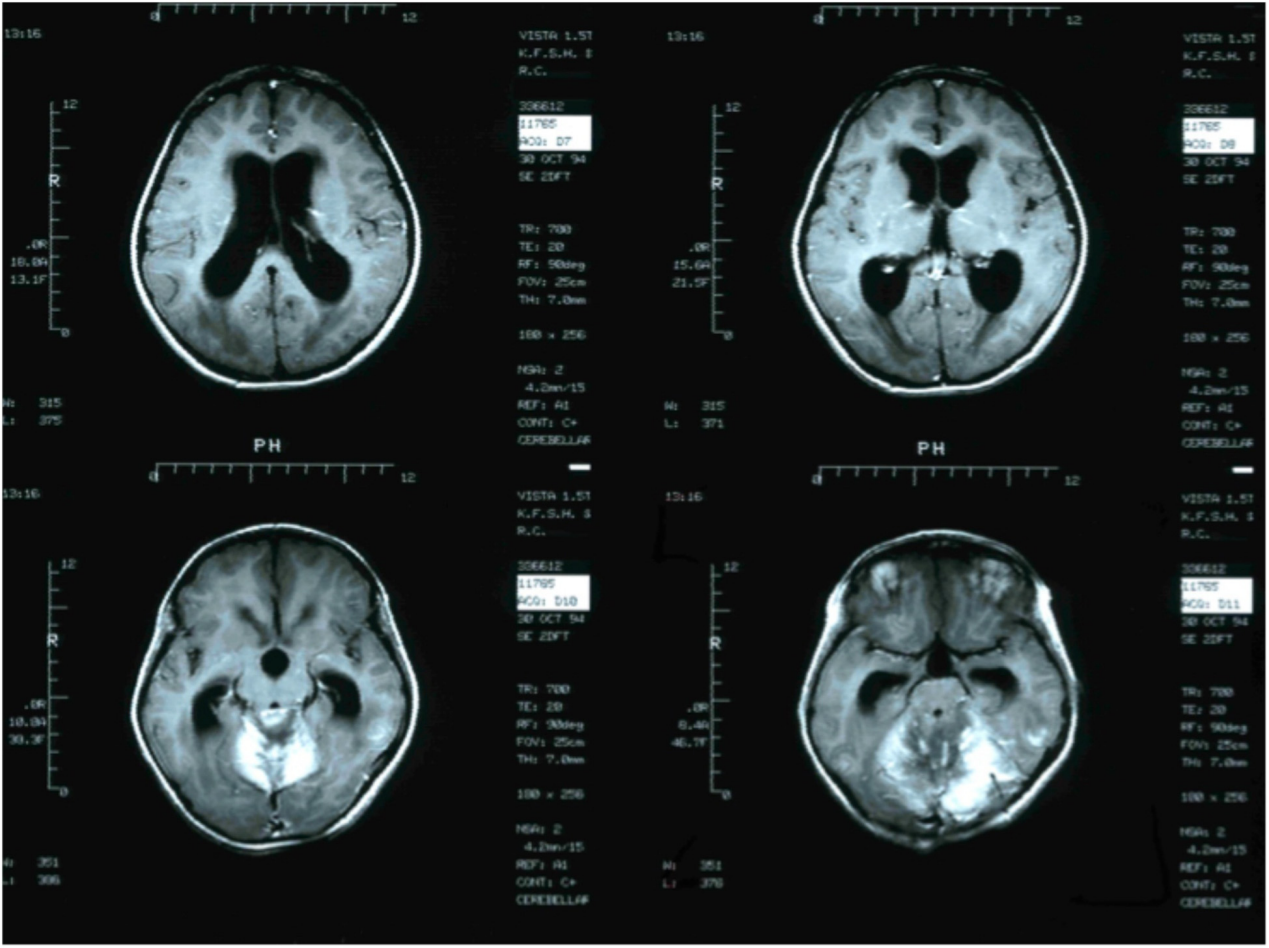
Brain MRI with contrast shows cerebellar enhancing lesions with secondary hydrocephalus.
